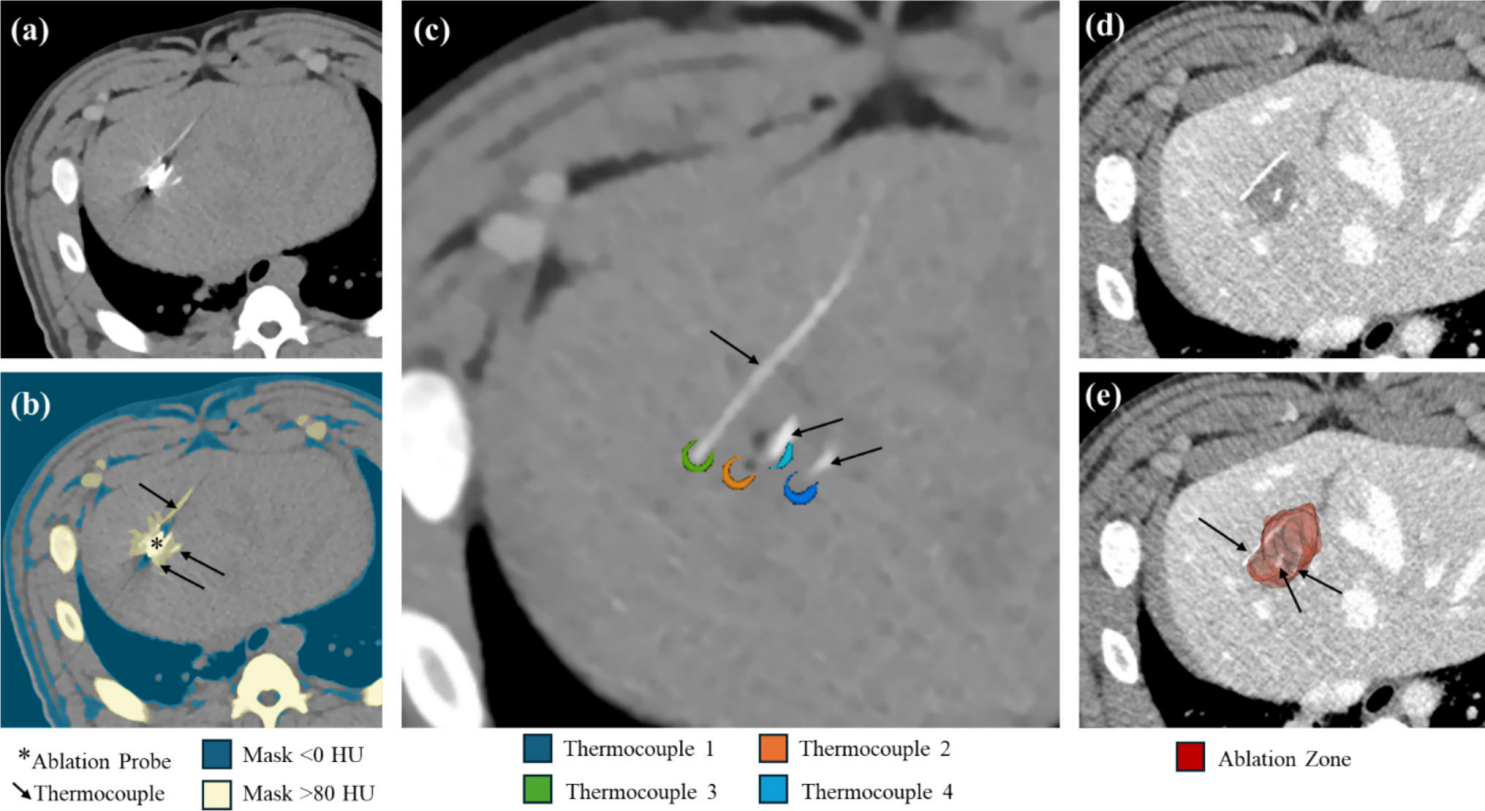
Image masks and segmentation. a A 70 keV VMI showing the ablation probe, three thermocouples and artifacts. b Masked areas of the ablation probe (asterisk), thermocouples (arrows), and artifacts. (c) Segmented regions of interest around the tips of thermocouples. d Contrast-enhanced CT showing the perfusion defect in the ablation zone and three thermocouples. e 3D segmentation of the ablation zone (red) shown in front of a single axial slice of the contrast-enhanced CT
